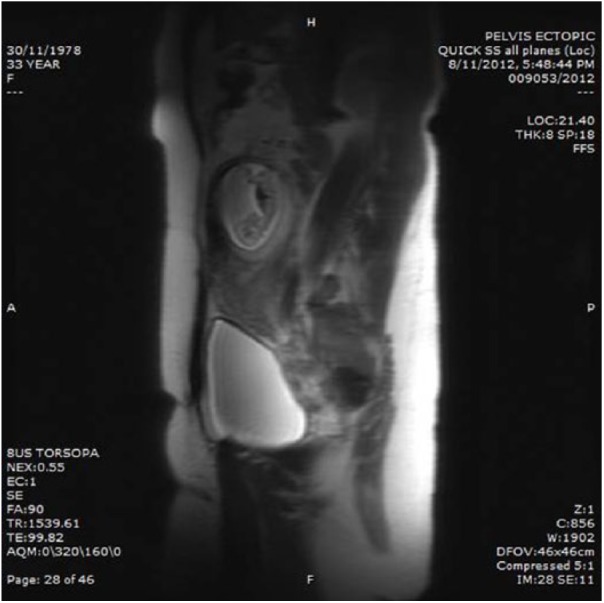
Sagittal views on magnetic resonance imaging of the abdomen demonstrating an
